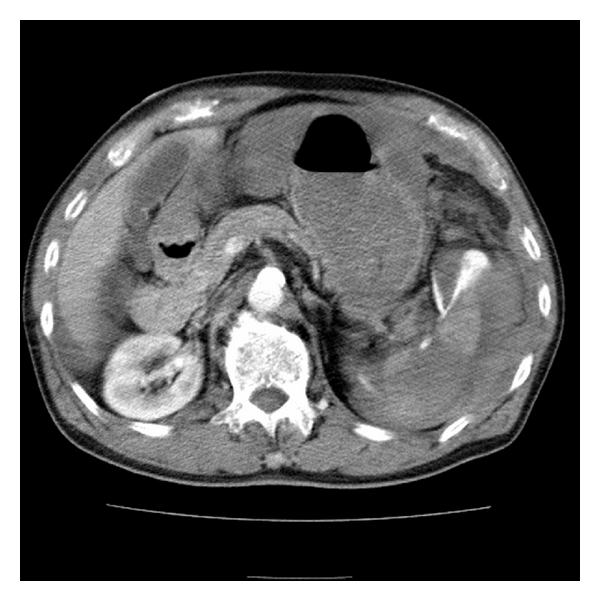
Transverse plane of helical CT of abdomen with IV contrast shows extravasation at splenic hilum and fluid around spleen.
